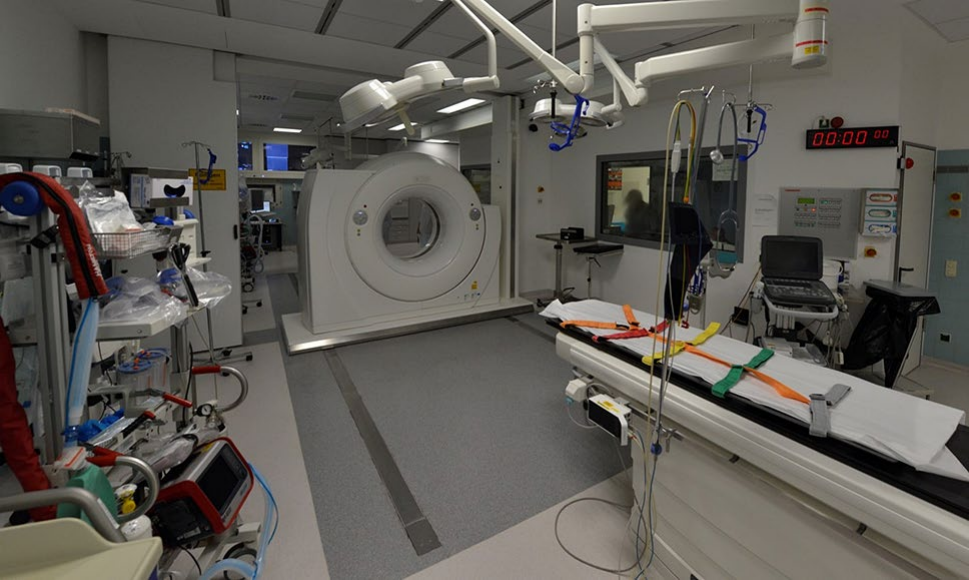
Overview of the trauma suite. The two trauma suites are equipped with a dual-room sliding gantry CT scanner in the middle of the two trauma suites. The rooms are separated by a radiation protection door. Both trauma suites are equipped equally
